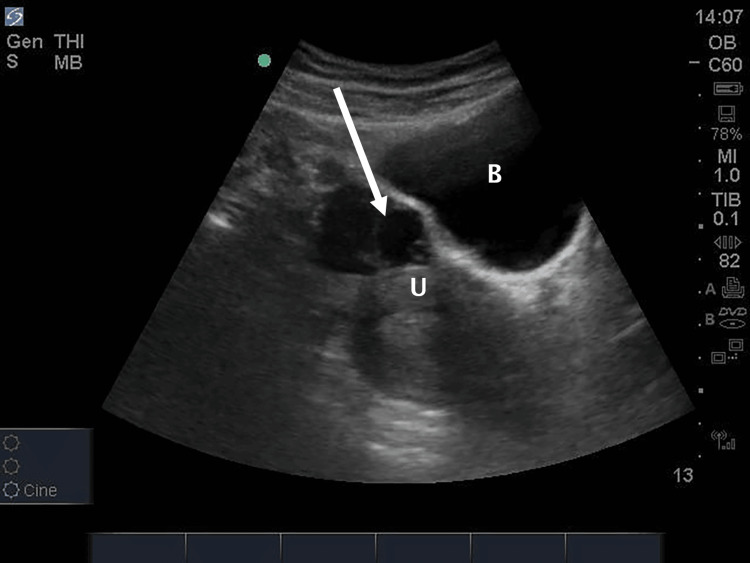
Transabdominal ultrasound exam showing a sagittal view of the pelvis. An anechoic cystic structure is seen within the left ovary with an additional internal ring-like structure, initially suspected to represent a yolk sac (arrow). The fundus of the uterus (U) and bladder (B) are also visualized.
